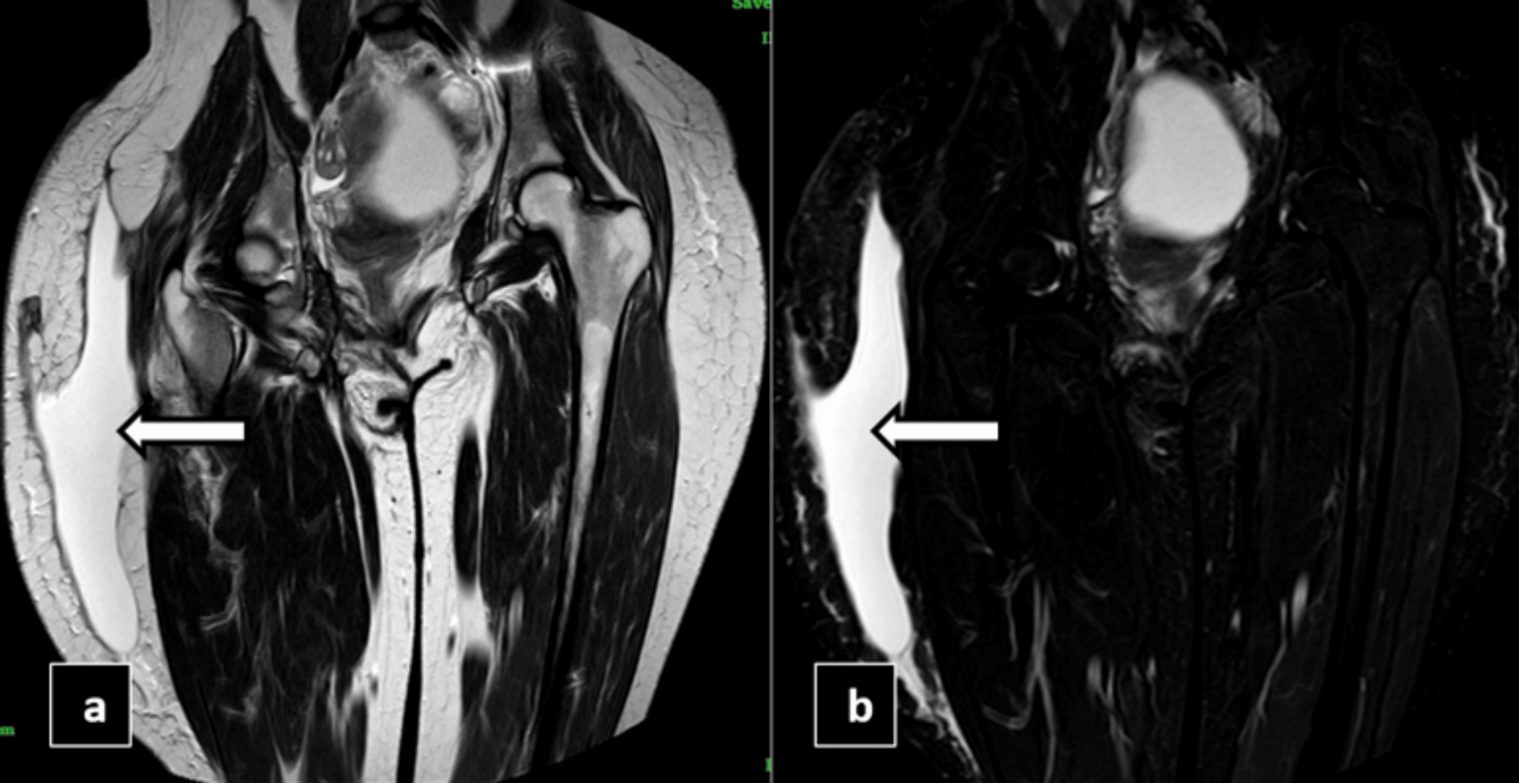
MRI T2 coronal reformatted image (a) and MRI short tau inversion recovery (STIR) coronal reformatted image (b) showing a fairly defined T2 and STIR hyperintense fluid collection noted between the subcutaneous and muscle layers of the right lateral aspect of the thigh region (white arrows).
